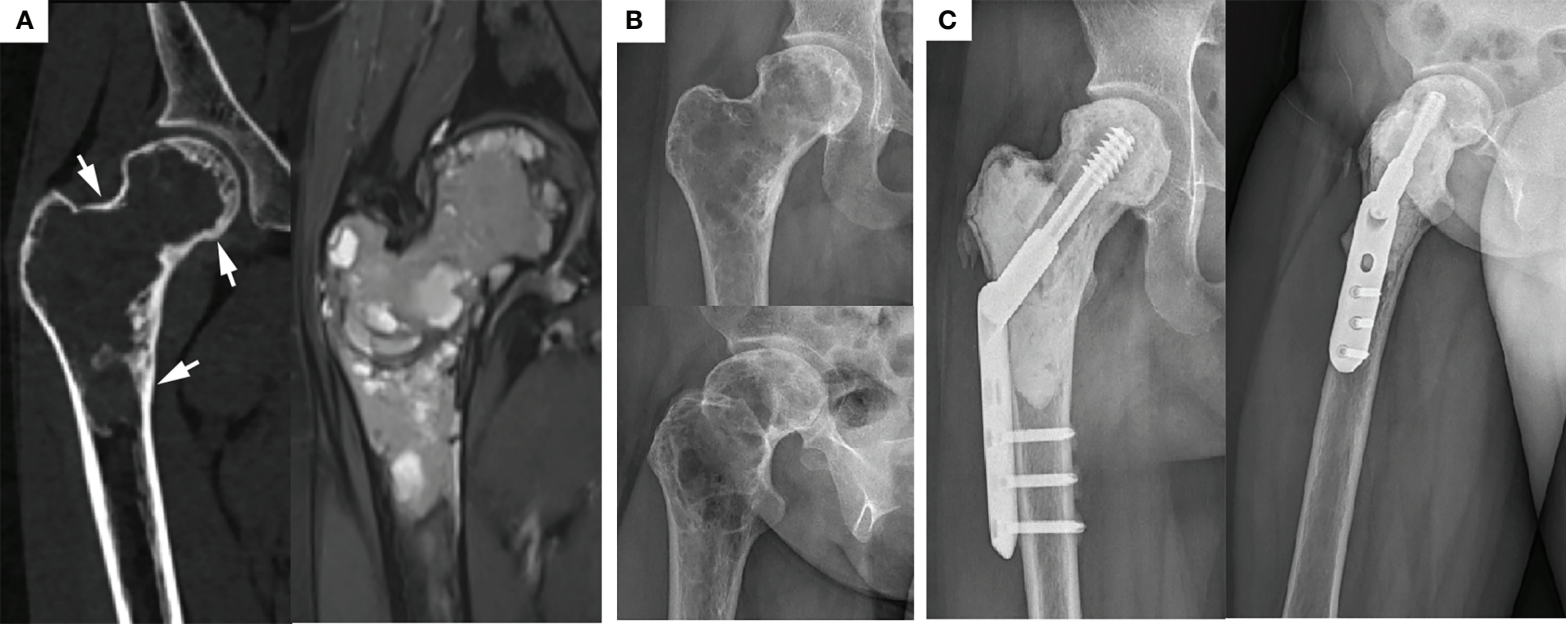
Typical preoperative and postoperative manifestations of EC for H1+H2+H3 type GCTB of proximal femur. (A) Preoperative CT and MRI showed that the femoral head, femoral neck, and subtrochanteric were invaded, but the lesions were still wrapped in the bone cortex (arrow). (B) Preoperative X-ray showed typical “soap bubble-like” changes. (C) Due to the extensive involvement of the lesion and the significant reduction of bone strength, the allogeneic fibula was placed in parallel above DHS and achieved a desirable prognosis 3 years after operation.
